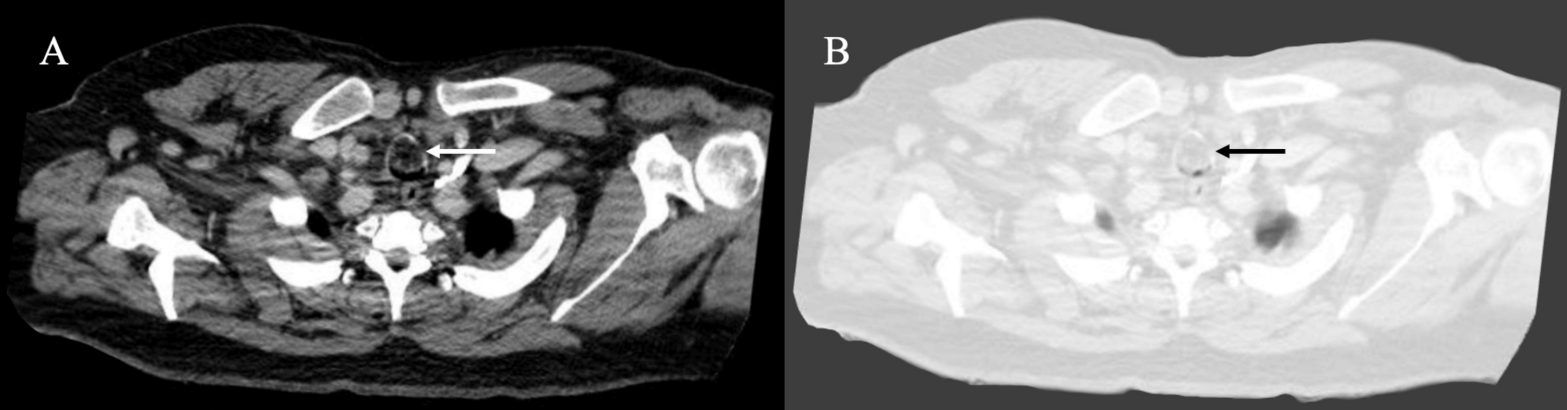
(A, B) Axial view of the CT revealing tracheal tumor in the abdomen and lung window, respectively (white and black arrows).
